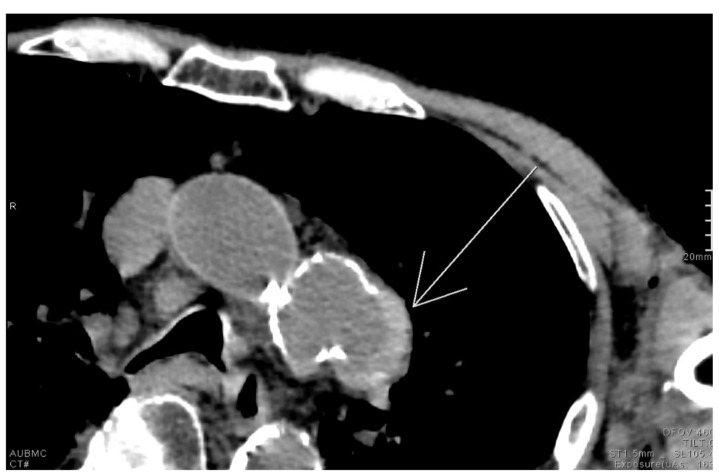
Unenhanced chest CT with findings of the hyperdense crescent sign noted along the left lateral wall of a saccular aneurysm arising from the distal aortic arch (white arrow).
